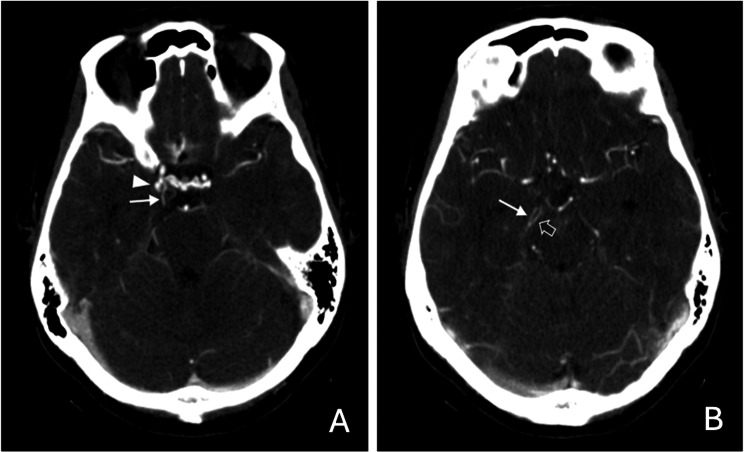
Consecutive axial CTA sections of the brain (A,B) The images show an aneurysm (arrowhead in A) at the junction of the right ICA and PCoA (arrows in A and B). The right PCoA extends posteriorly as a prominent vessel running parallel to the classical right PCA arising from the basilar trunk (open arrow in B) toward the occipital lobe. CTA: Computed tomography angiography, ICA: Internal carotid artery, PCoA: Posterior communicating artery, PCA: Posterior cerebral artery
